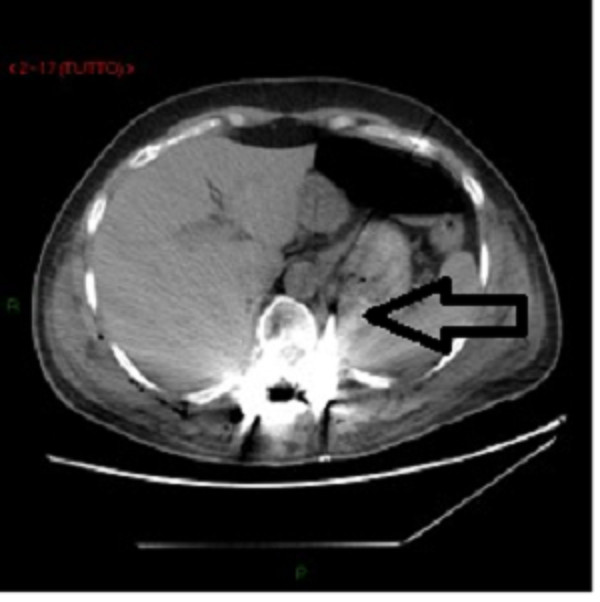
CT scan. Black arrow indicates the misplaced pedicle screw.
